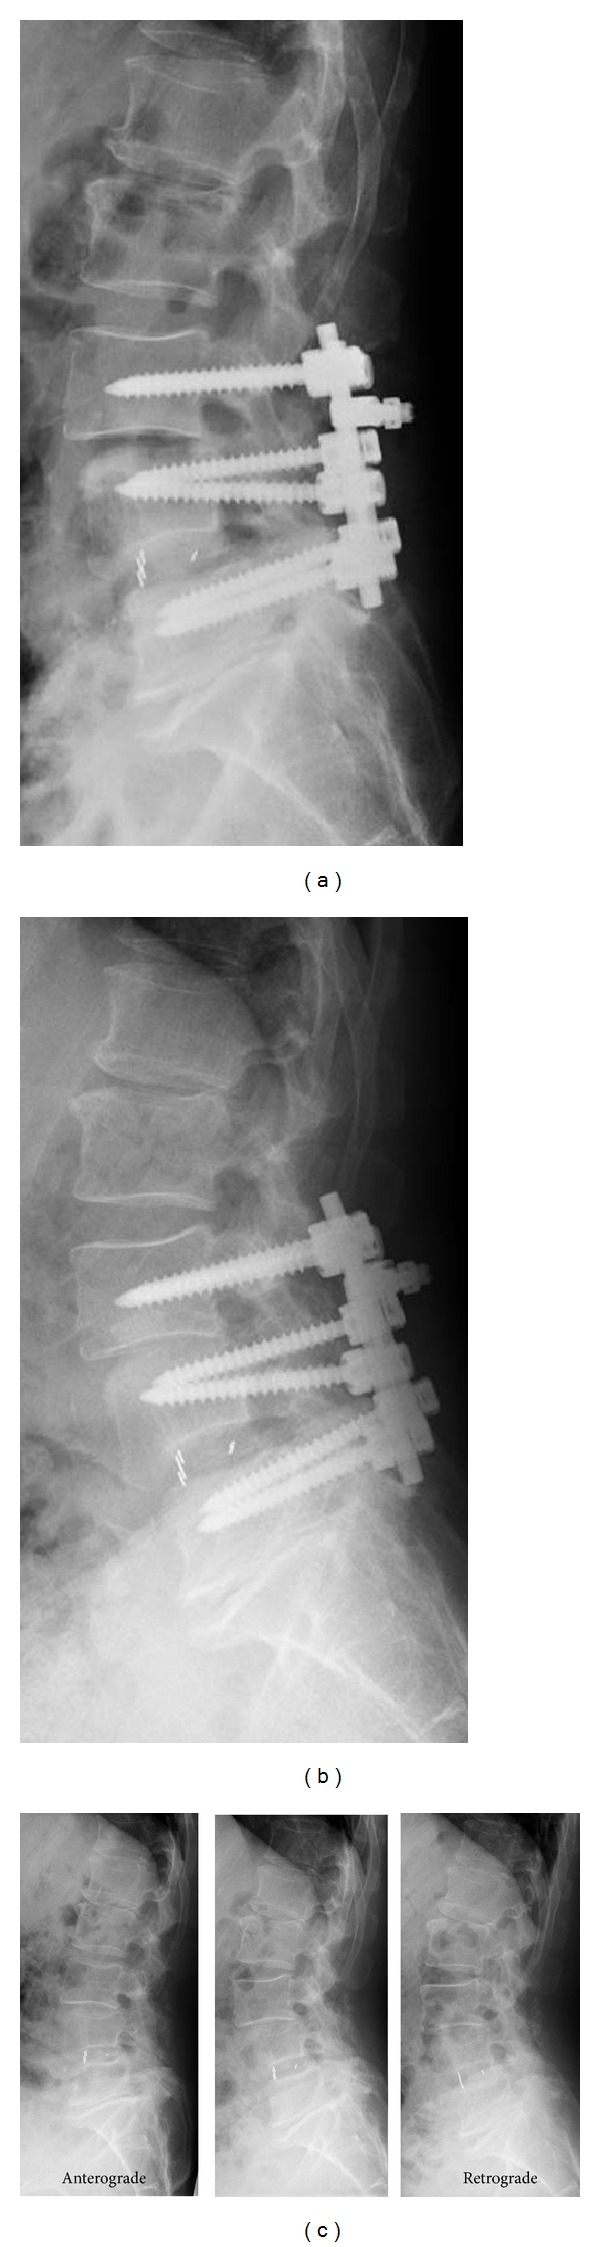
Plain lumbar radiographs taken just after the fusion operation (a), 1 year after the operation (b), and 2 years after the operation (c). There was slight retrolisthesis and decreased posterior disc height on L2/3 on 1-year (b) and 2-year follow-up (c), but with no definite instability (c).
